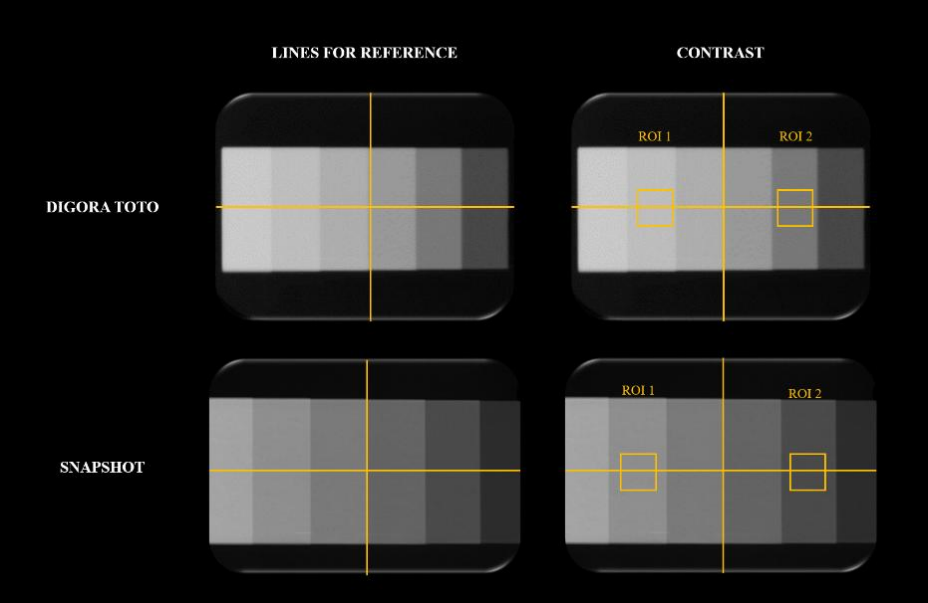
Radiographs with vertical and horizontal yellow lines as references were used to place ROIs 1 and 2 for contrast evaluation. *ROI, regionof interest*
